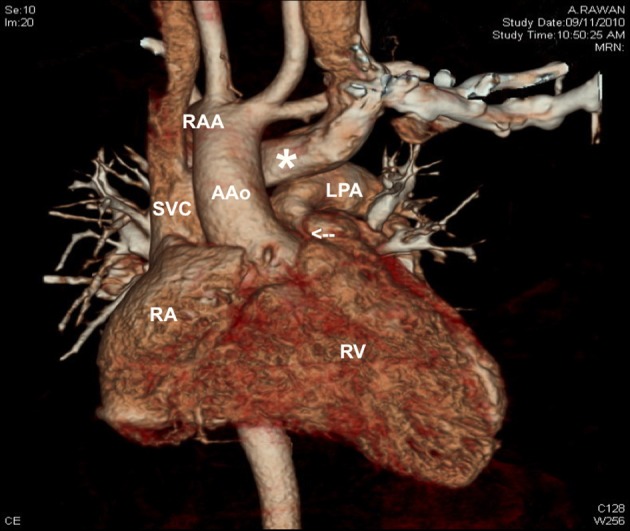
CT angiography scan showing the presence of retro-aortic innominate vein (white asterisk), in a patient with tetralogy of Fallot with narrowed infundibulum (white arrow) and right aortic arch. AAo, ascending aorta; LPA, left pulmonary artery; RAA, right aortic arch; RA, right atrium; RV, right ventricle; SVC, superior vena cava.
